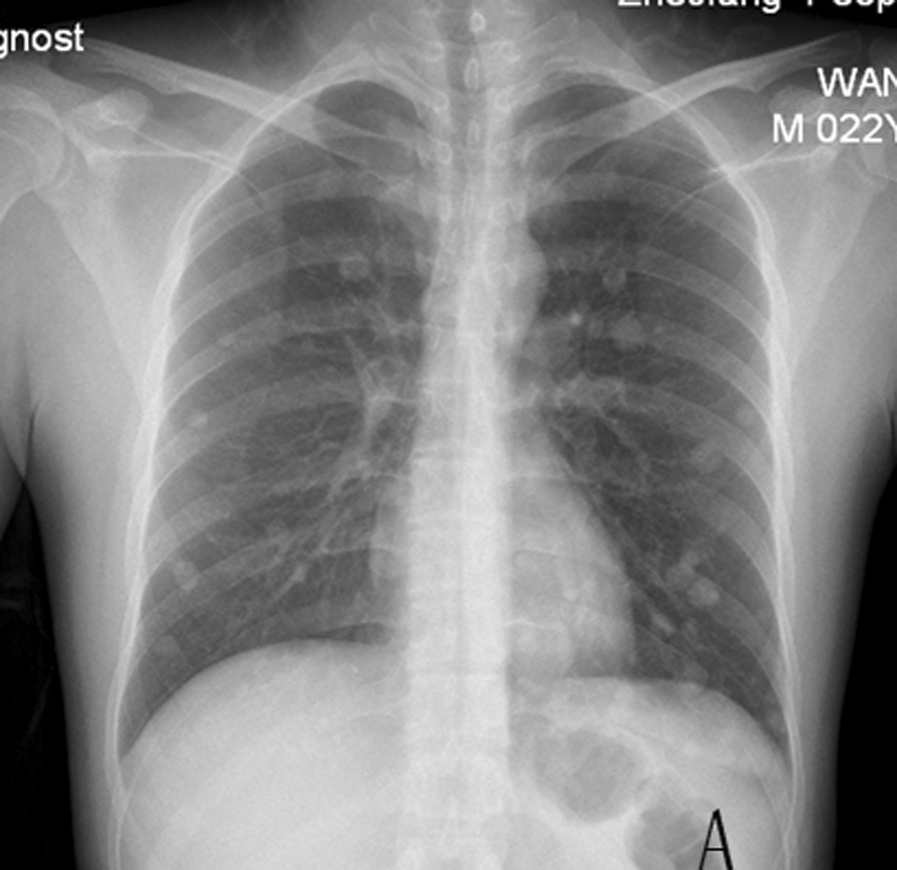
Chest radiography. (A) Evidence of lung metastasis.
